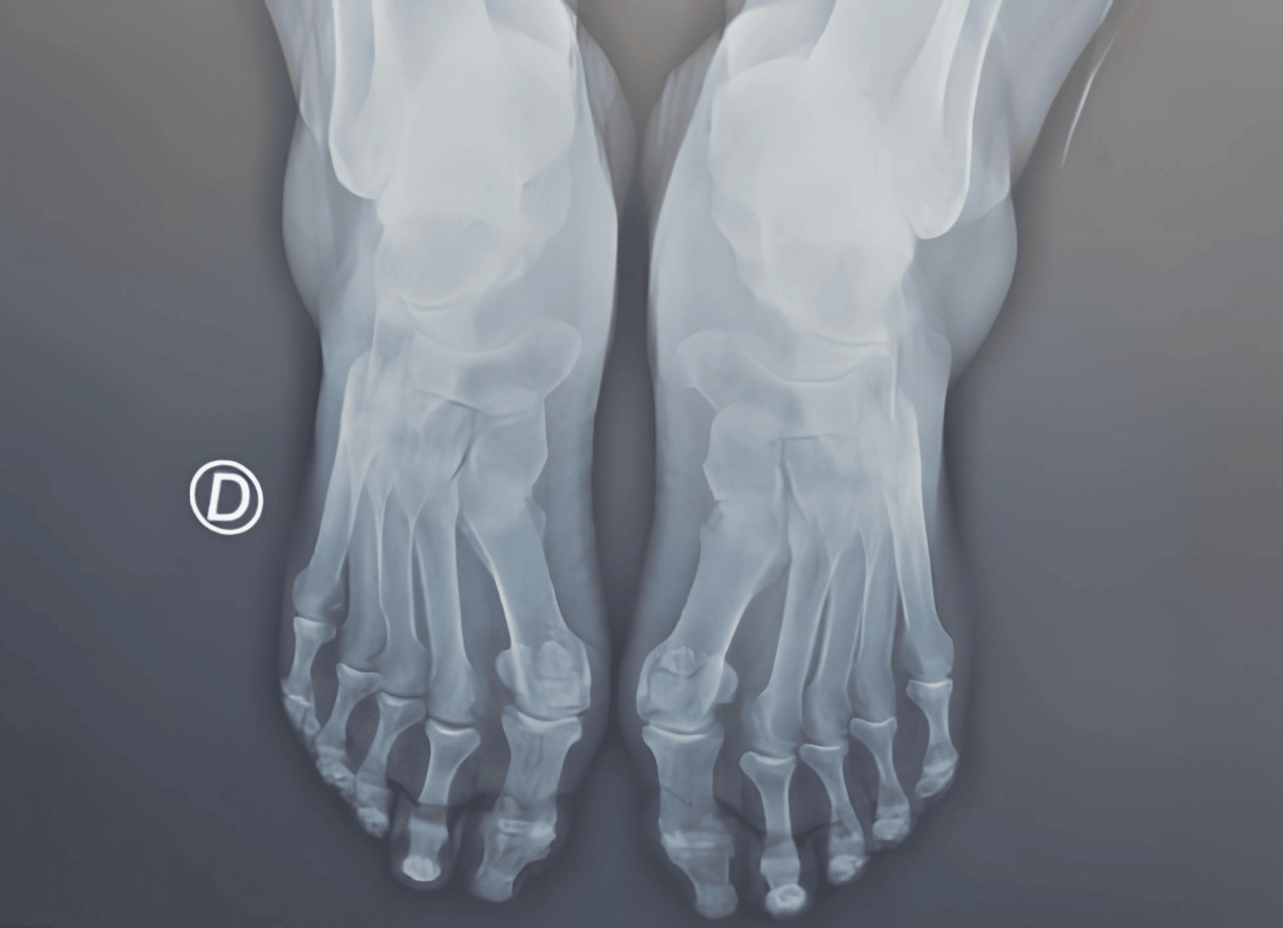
Bilateral hallux valgus X-ray. D: right foot.
